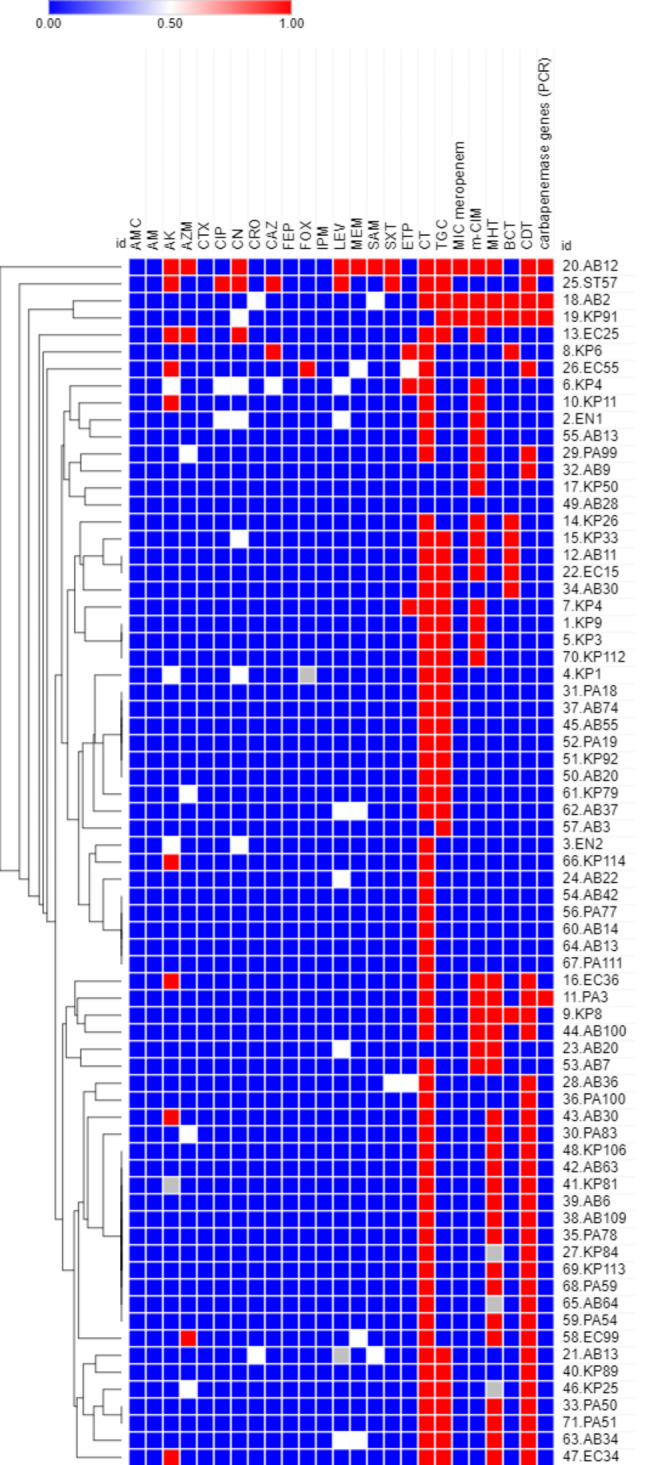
The heat-map clonal analysis of the among the recovered carbapenem-resistance Gram-negative bacilli (CR-GNB) phenotypes. AMC (amoxicillin/clavulanic acid), AM (ampicillin), AK (amikacin), AZM (aztreonam), CTX (cefotaxime), CIP (ciprofloxacin), CRO (ceftriaxone), CAZ (ceftazidime), FEP (cefepime), FOX (cefoxitin), (CT) colistin, ETP (ertapenem), CN (gentamicin), IPM (imipenem), LEV (levofloxacin), (MEM) meropenem), (SAM) ampicillin/ sulbactam, SXT (trimethoprim / sulfamethoxazole, TGC (tigecycline). MHT (modified Hodge test), mCIM (modified carbapenem inactivation method), CDT, (combined disk test by EDTA), BCT (blue-carba test)
